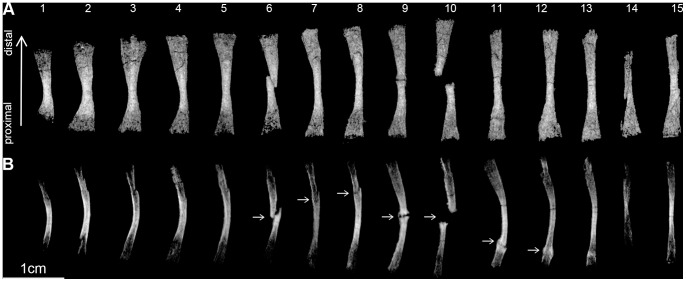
Right rib series of the *Broomistega putterilli*. A, 3D rendering of the rib series in dorsal view with individual ribs numbered from anterior to posterior (1 to 15). B, virtual radiography of the rib series in anterior view. The series of ribs display ante-mortem fractures (white arrows in B), some of which exhibit healing (ribs 9 to 12), including pseudoarthroses.
